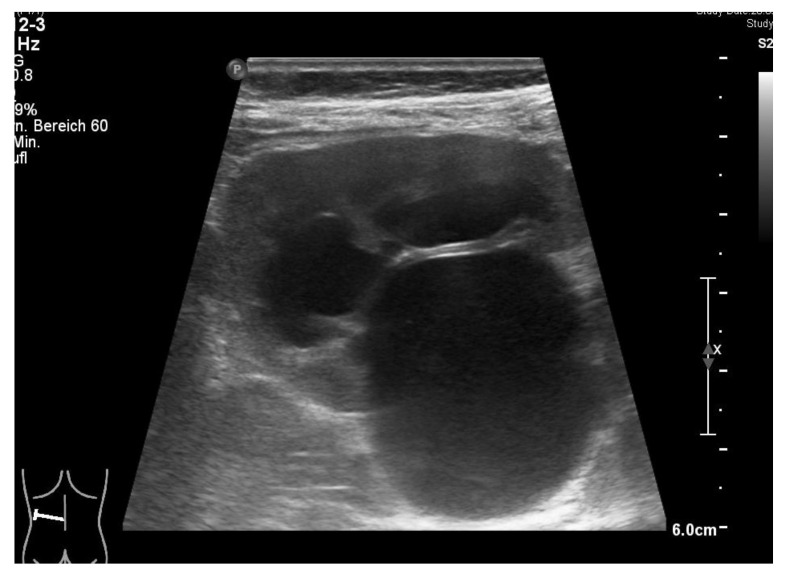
Ultrasound test of 1.5-year-old girl (19 May 2020) presenting with recurrent febrile urinary tract infections shows dilatation of the pyelon and hydronephrosis of the left kidney (courtesy of Dr. A. Longin, Klinikum Esslingen).
